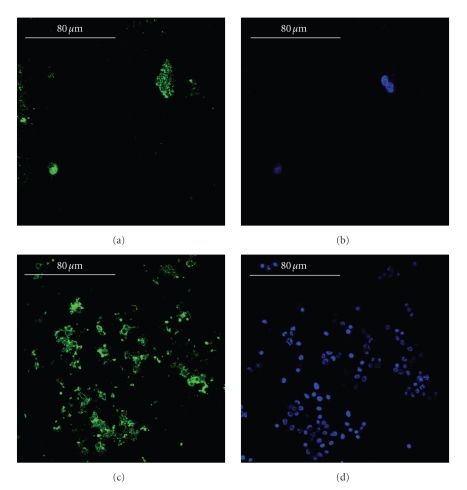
Immunolocalization of decorin (panels a and c, green) and cellular nuclei (panels b and d, blue) in the static culture (panels a and b) and in the ultrasonic culture (panels c and d), bars equal to 80 *μ*m. During the culture period, in the control (panels a and b), the osteoblasts produced a very little amount of decorin, a key regulator for matrix spatial organization, whereas, in the stimulated culture (panels c and d), the osteoblasts secreted a larger amount of 3D organized bone matrices.
